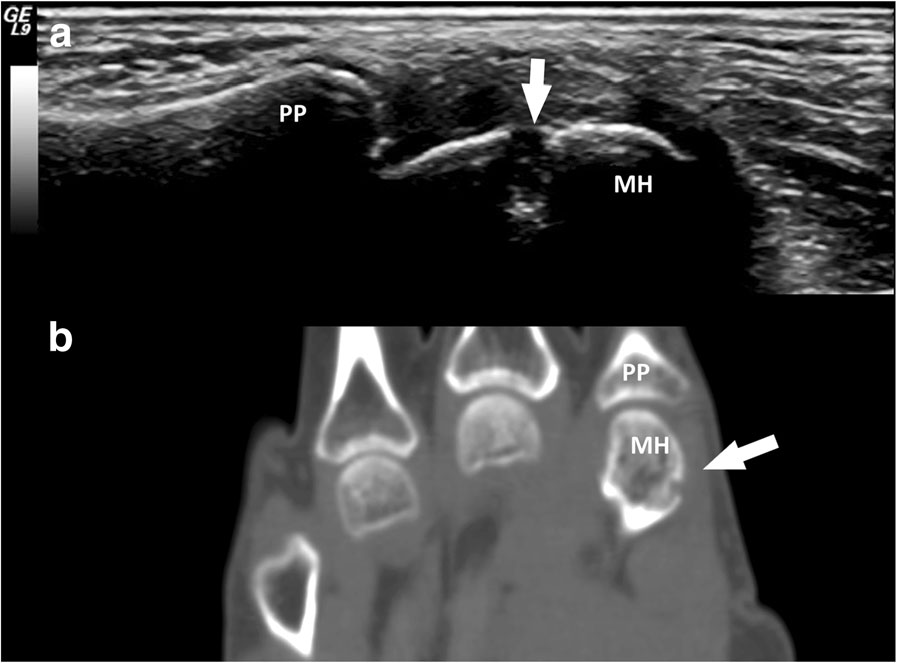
Bone erosions in a patient with rhupus syndrome. Longitudinal ultrasound image of the second metacarpophalangeal joint lateral area showing a grade-2 scoring ultrasound structural erosion (*arrow*) with sharp margins (a) corresponding to a grade-2 rheumatoid arthritis magnetic resonance imaging score erosion (*arrow*) visible in the computed tomography coronal scan of the hand (b). *MH* second metacarpal head, *PP* second proximal phalanx
